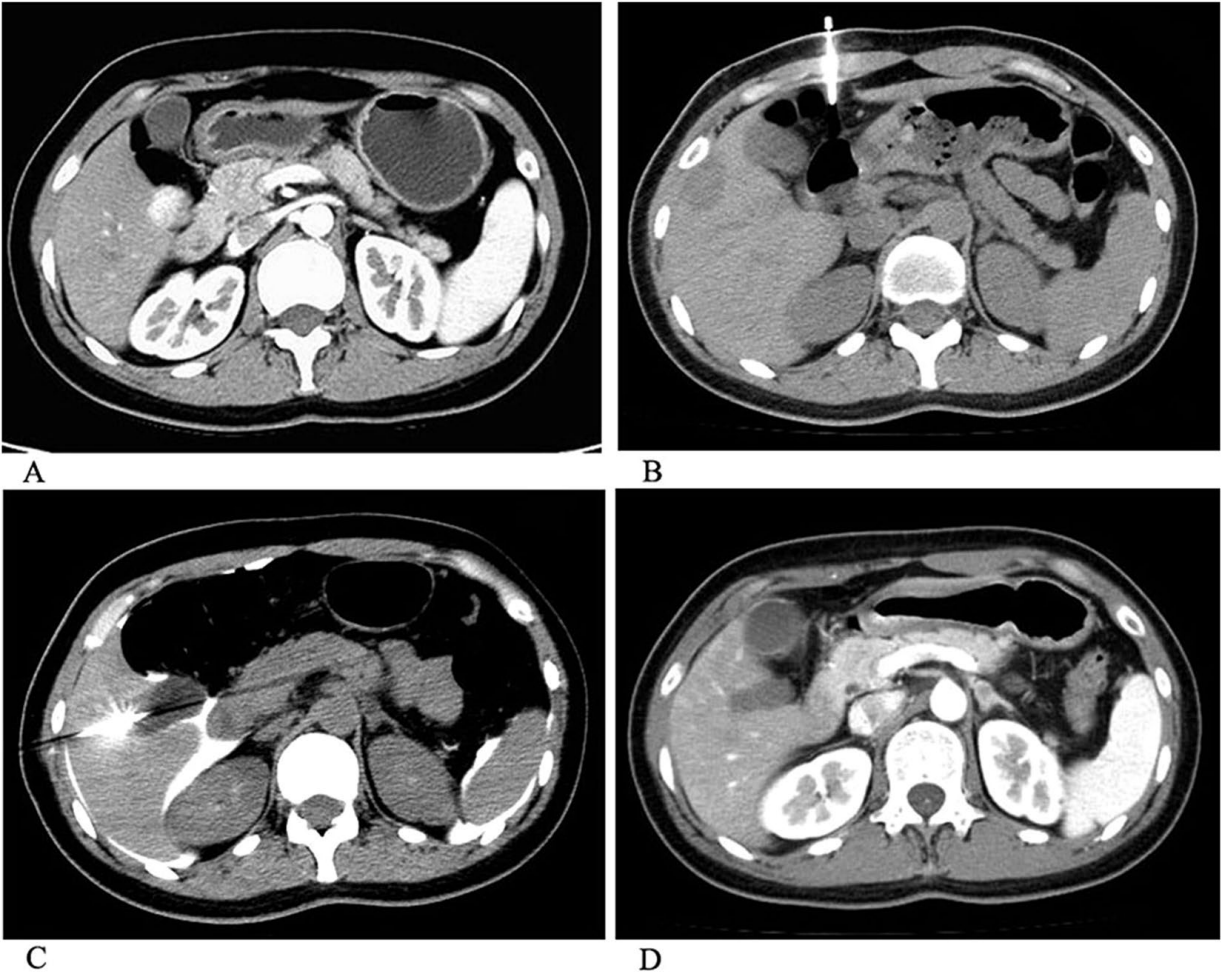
Artificial ascites assisting percutaneous cryoablation of a hepatic adenoma adjacent to the duodenum in a 34 year old female. (A) CT demonstrates a hepatic adenoma adjacent to the duodenum. (B) A 20-gauge introducer needle was inserted into the peritoneal cavity along the edge of the liver under CT guidance. (C) Then, a sufficient amount of 2.0% iodinated contrast was injected; we see the artificial ascites separates the tumor and adjacent duodenum. Two freeze/thaw cycles (12.0 min freeze, 3.0 min passive thaw) were applied. CT demonstrates the ice ball covering the tumor completely. (D) Contrast-enhanced CT showed tumor was complete ablation after 3 months followed up.
